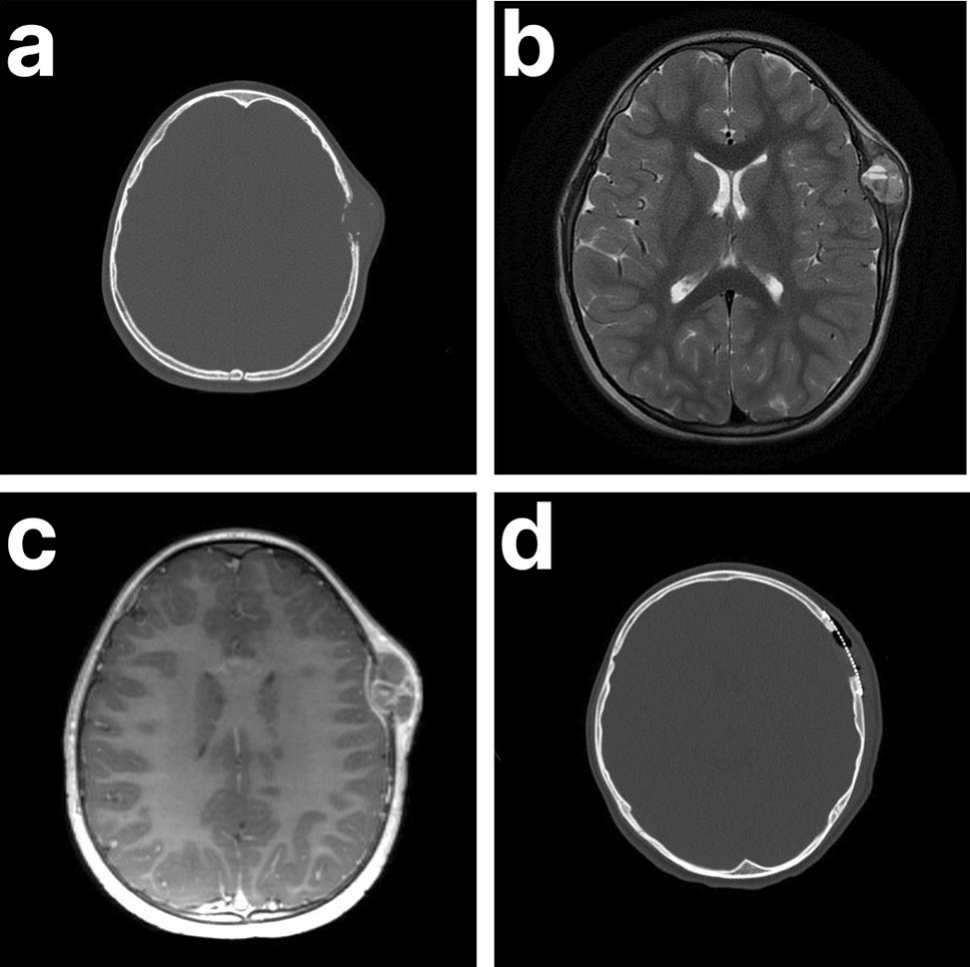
Panel a: CT scan shows an expansile, destructive lesion of the left parietal bone. Panel b: T2-weighted MRI demonstrates a well-defined, lytic lesion with internal septations and fluid-fluid levels. Panel c: Post-contrast T1-weighted MRI demonstrates epidural enhancement adjacent to the lesion, with no absolute evidence of dural involvement or invasion. Panel d: Post-operative CT demonstrating that the skull lesion was surgically resected and a titanium mesh cranioplasty was performed. *Abbreviations: CT, computed tomography; MRI, magnetic resonance imaging*
